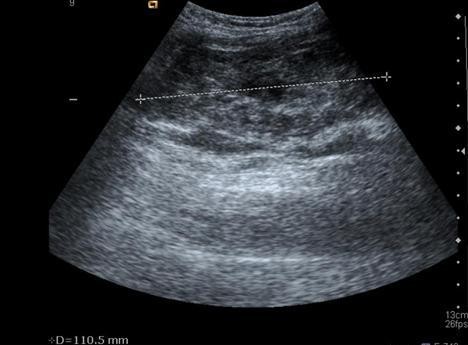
Ultrasound. Ultrasound showing an abscess with gas inside, located in the right thigh.
